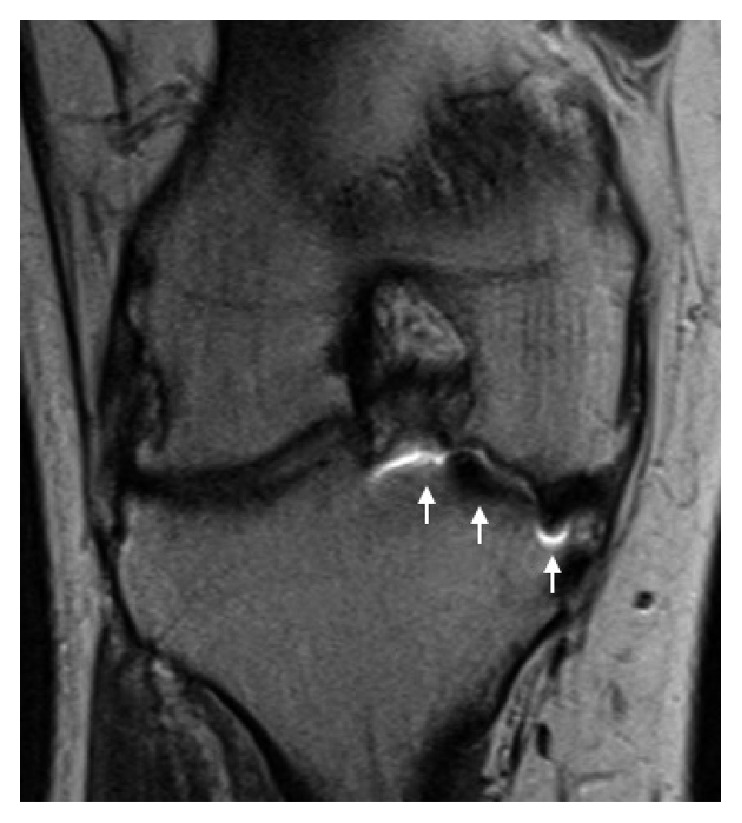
Coronal proton density with metal reduction MRI of the right knee demonstrates significant image distortion (white arrows) from metallic artifacts, obscuring accurate evaluation of the medial femoral condyle articular surface.
